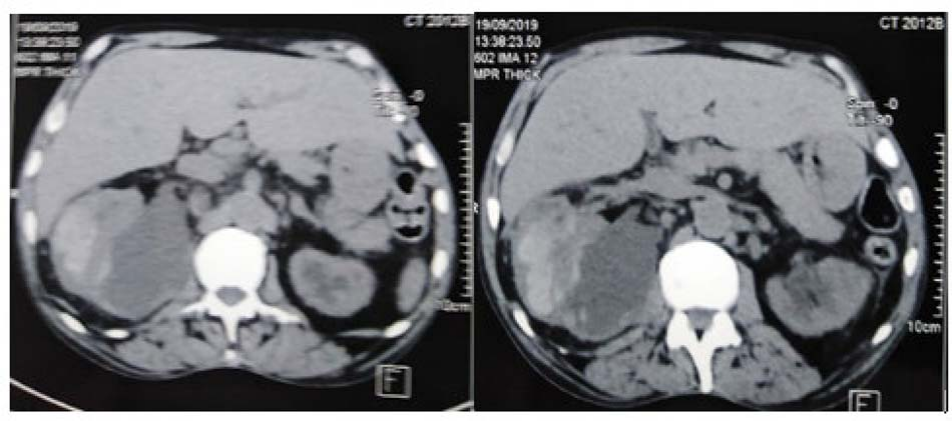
The CT scan showed a huge subcapsular hematoma (12 × 14 × 10 cm) of the right kidney.
